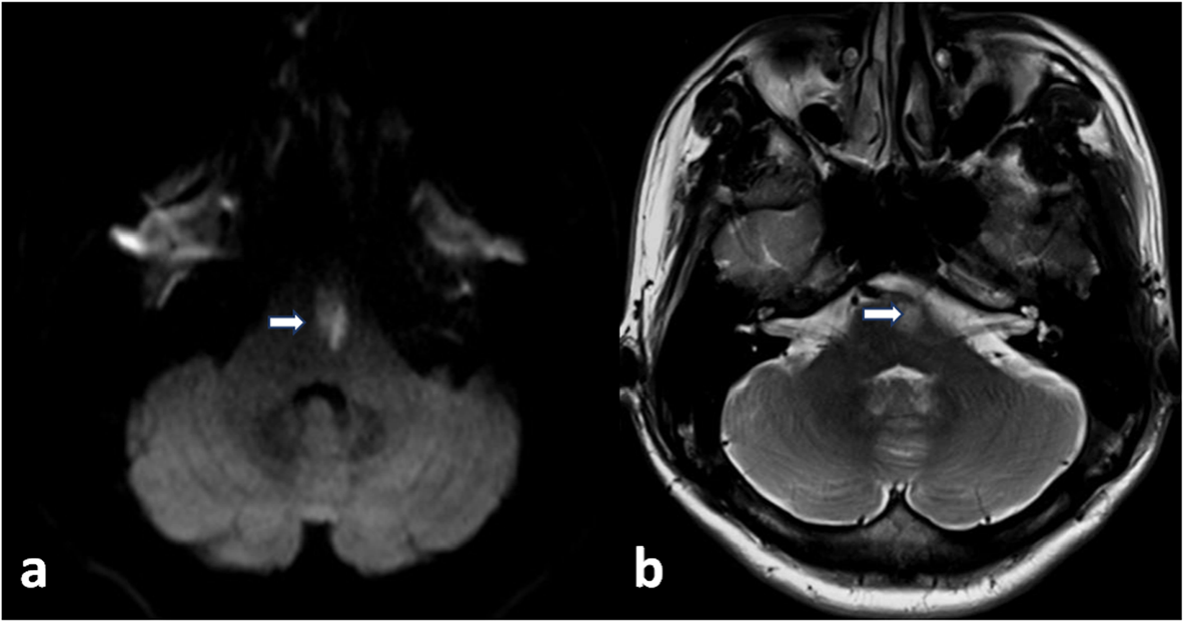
Diffusion weighted imaging (a) and T2-weighted image (b) showed pons infarction (arrow)
